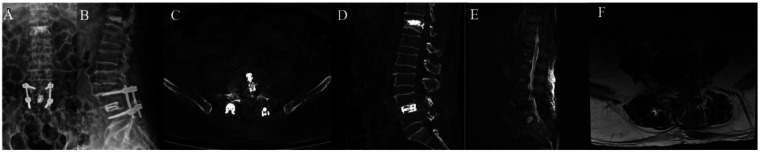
Postoperative imaging. (A) A/P and (B) lateral radiographs showing the expandable cage at L4–L5. (C) Axial and (D) sagittal CT images confirming correct cage position. (E) Sagittal and (F) axial MRI showing no significant spinal cord compression or cerebrospinal fluid leakage.
